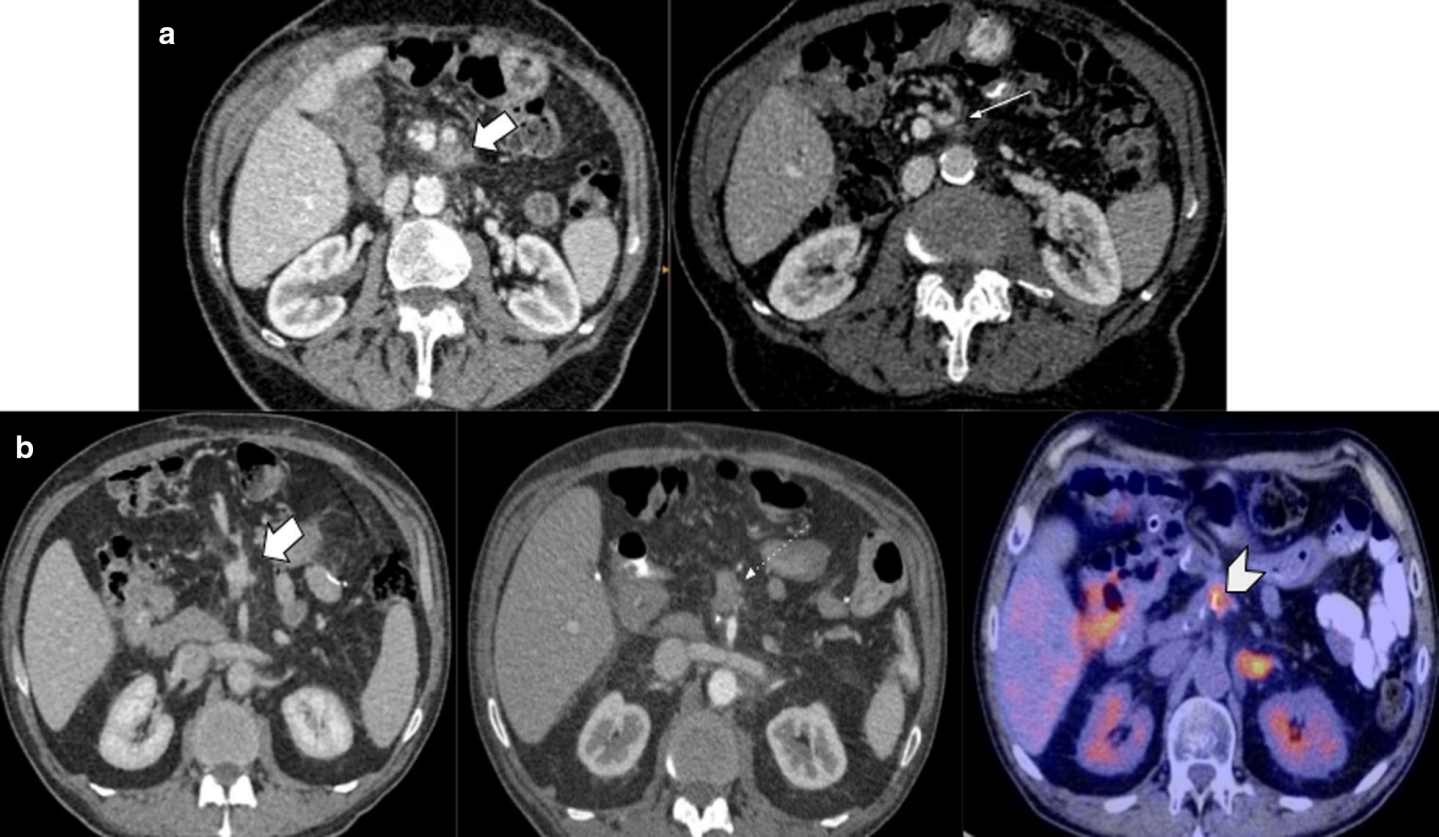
Perivascular cuffing vs. tumor recurrence. CT challenge. Two different patients (a) 81-year-old male patient three weeks after pancreaticoduodenectomy due to ampulloma. (b) 45-year-old male patient with fever 21 days after pancreaticoduodenectomy due to adenocarcinoma. Soft-tissue imaging in the surgical bed (thick white arrows), in both cases strict follow-up was required. In patient A, the focal fat stranding has markedly resolved (thin arrow): while in patient B persists without significant change after a year (dotted arrow). PET/CT shows hypercaptation mass, which confirms tumor recurrence (arrowhead)
